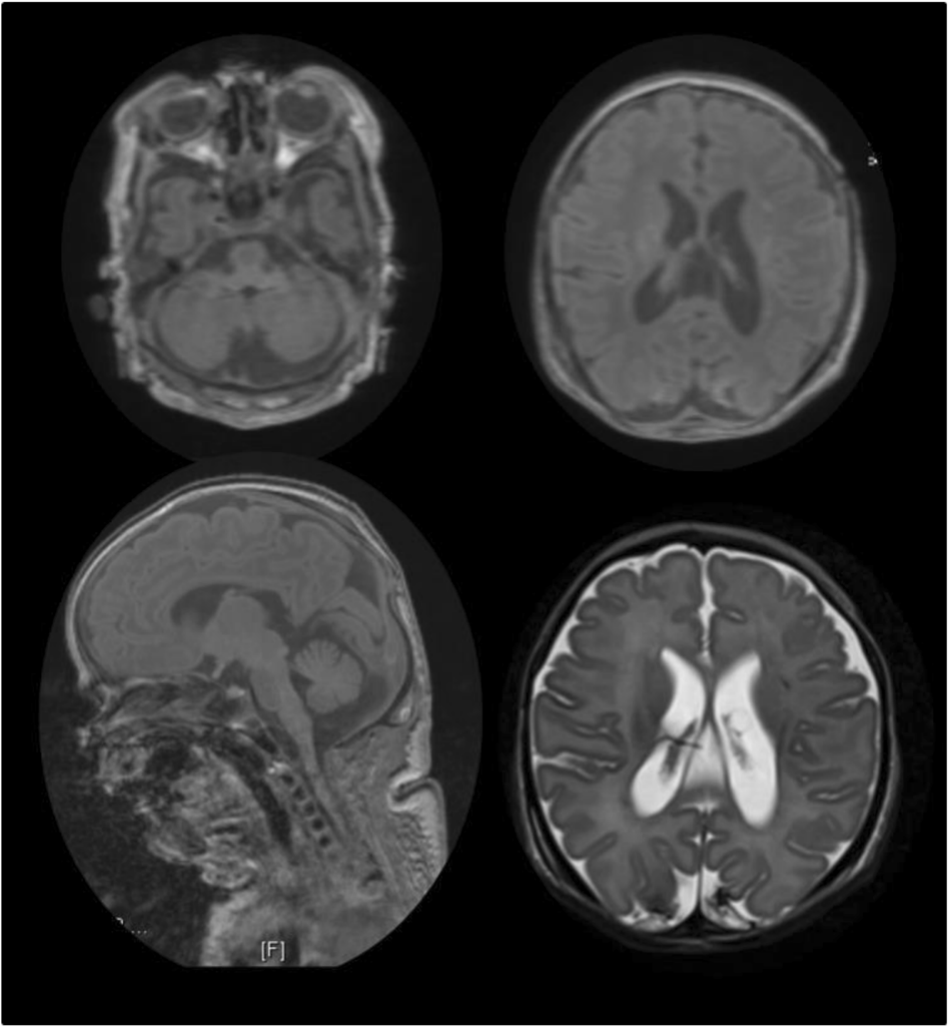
Magnetic resonance imaging (MRI). Cranial and thoracic MRI performed at the age of 6 weeks revealed a hypoplastic cerebellum, necrosis and haemorrhage of the occipital cortex, pronounced atrophy of skeletal musculature and confirmed bilateral pleural effusion.
